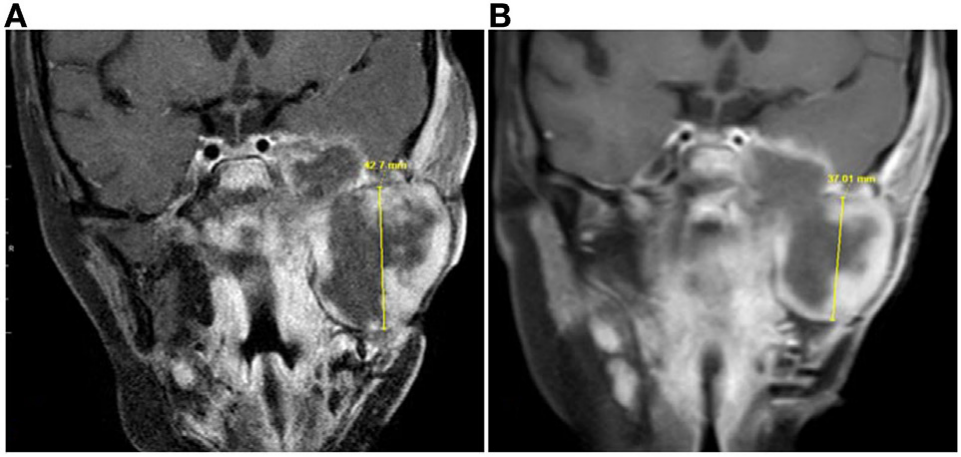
Magnetic resonance imaging (MRI) taken prior to and during vorinostat treatment. Coronal contrast-enhanced T1-weighted MRI images from (A) July 2014 and (B) September 2014 showed decrease in size of the infratemporal component of the tumor. Craniocaudal measurement showed the greatest change, although this component of the tumor had decreased in size in other dimensions as well.
